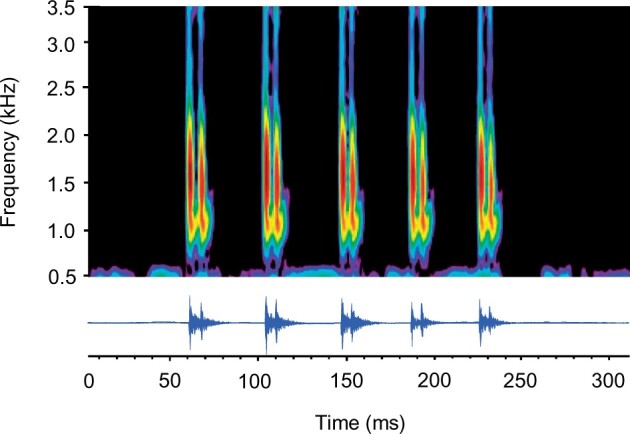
Sonogram (above) and oscillogram (below) of a croaking sound of *Trichopsis vittata*, consisting of 5 double-pulsed bursts. Main energies are between 1 and 2 kHz, pictured by the dark red color which shows the highest energy level (sampling frequency 44.1 kHz, filter bandwidth 250 Hz, 75% overlap, Hanning window).
